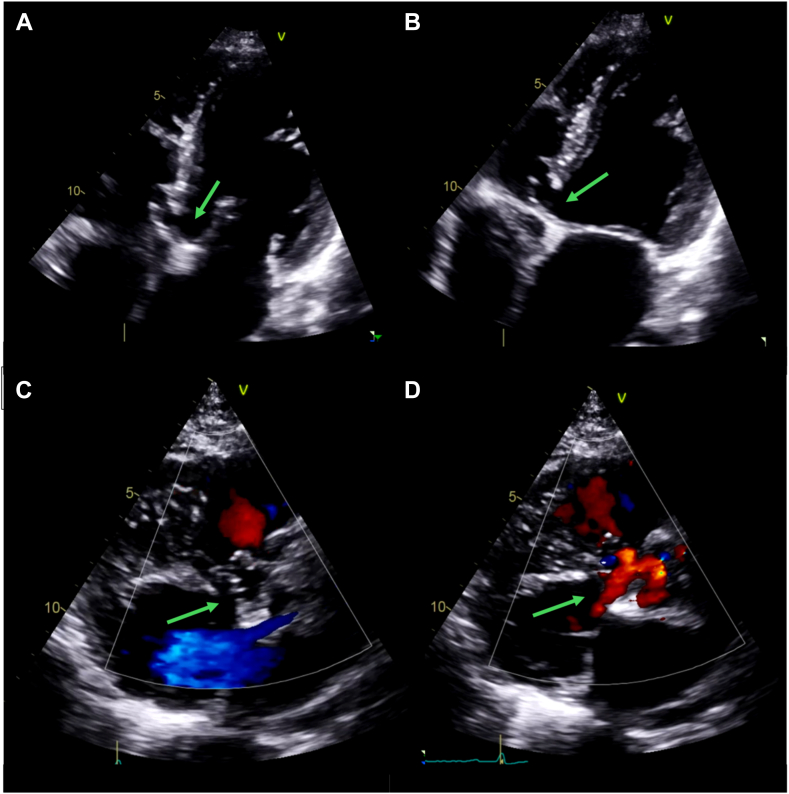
Two-dimensional TTE without (*top*) and with (*bottom*) color-flow Doppler, modified apical 4-chamber (A and B) and parasternal long-axis (C and D), diastolic (A, C), and systolic (B, D) views, demonstrates a narrow-necked saccular outpouching anatomic defect that arises from the membranous septum, extends into the right ventricle adjacent to the septal tricuspid leaflet, is noncontracting, and is without evidence of left-to-right shunt, consistent with an IVMSA (*arrows*).
